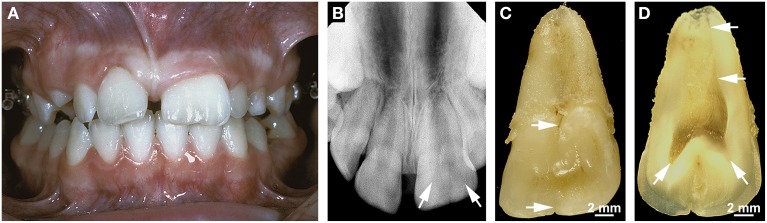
Clinical, radiographic, and macroscopic appearance of gemination. (A) An intraoral view of a geminated permanent maxillary left central incisor shows the size of the double tooth in comparison with that of the normal right central incisor. Note that the tooth count is correct if the oversized incisor is counted as one. (B) The apical radiograph from the same incisor reveals two faintly visible separate pulp horns (arrows). (C) A lingual macroscopic view of the double tooth shows a small notch in the incisal edge and a groove in the cervical tubercle (arrows) which continues as a shallow groove on the root. (D) A macroscopic view of the mesio-distally cut incisor demonstrates the partially divided coronal pulp and the single root canal (arrows). Original magnifications (C,D) 4x.
